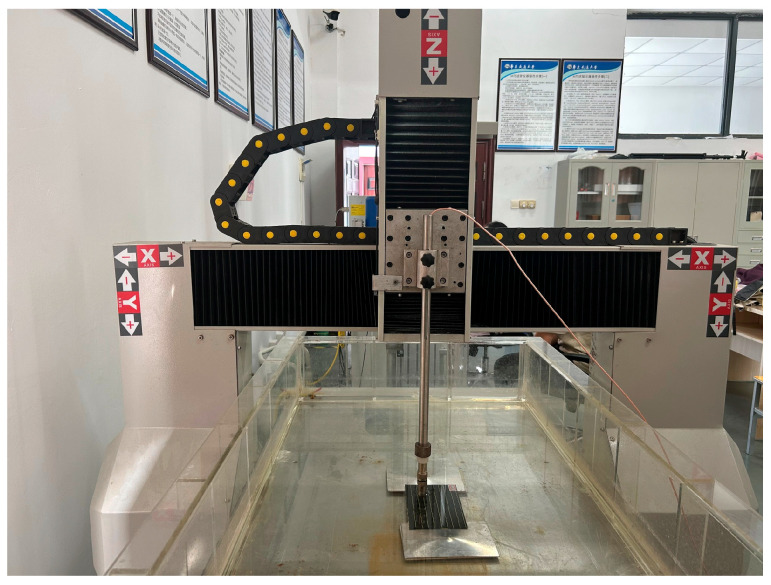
Water-immersed ultrasonic C-scan device UTScanMaster-6090.
